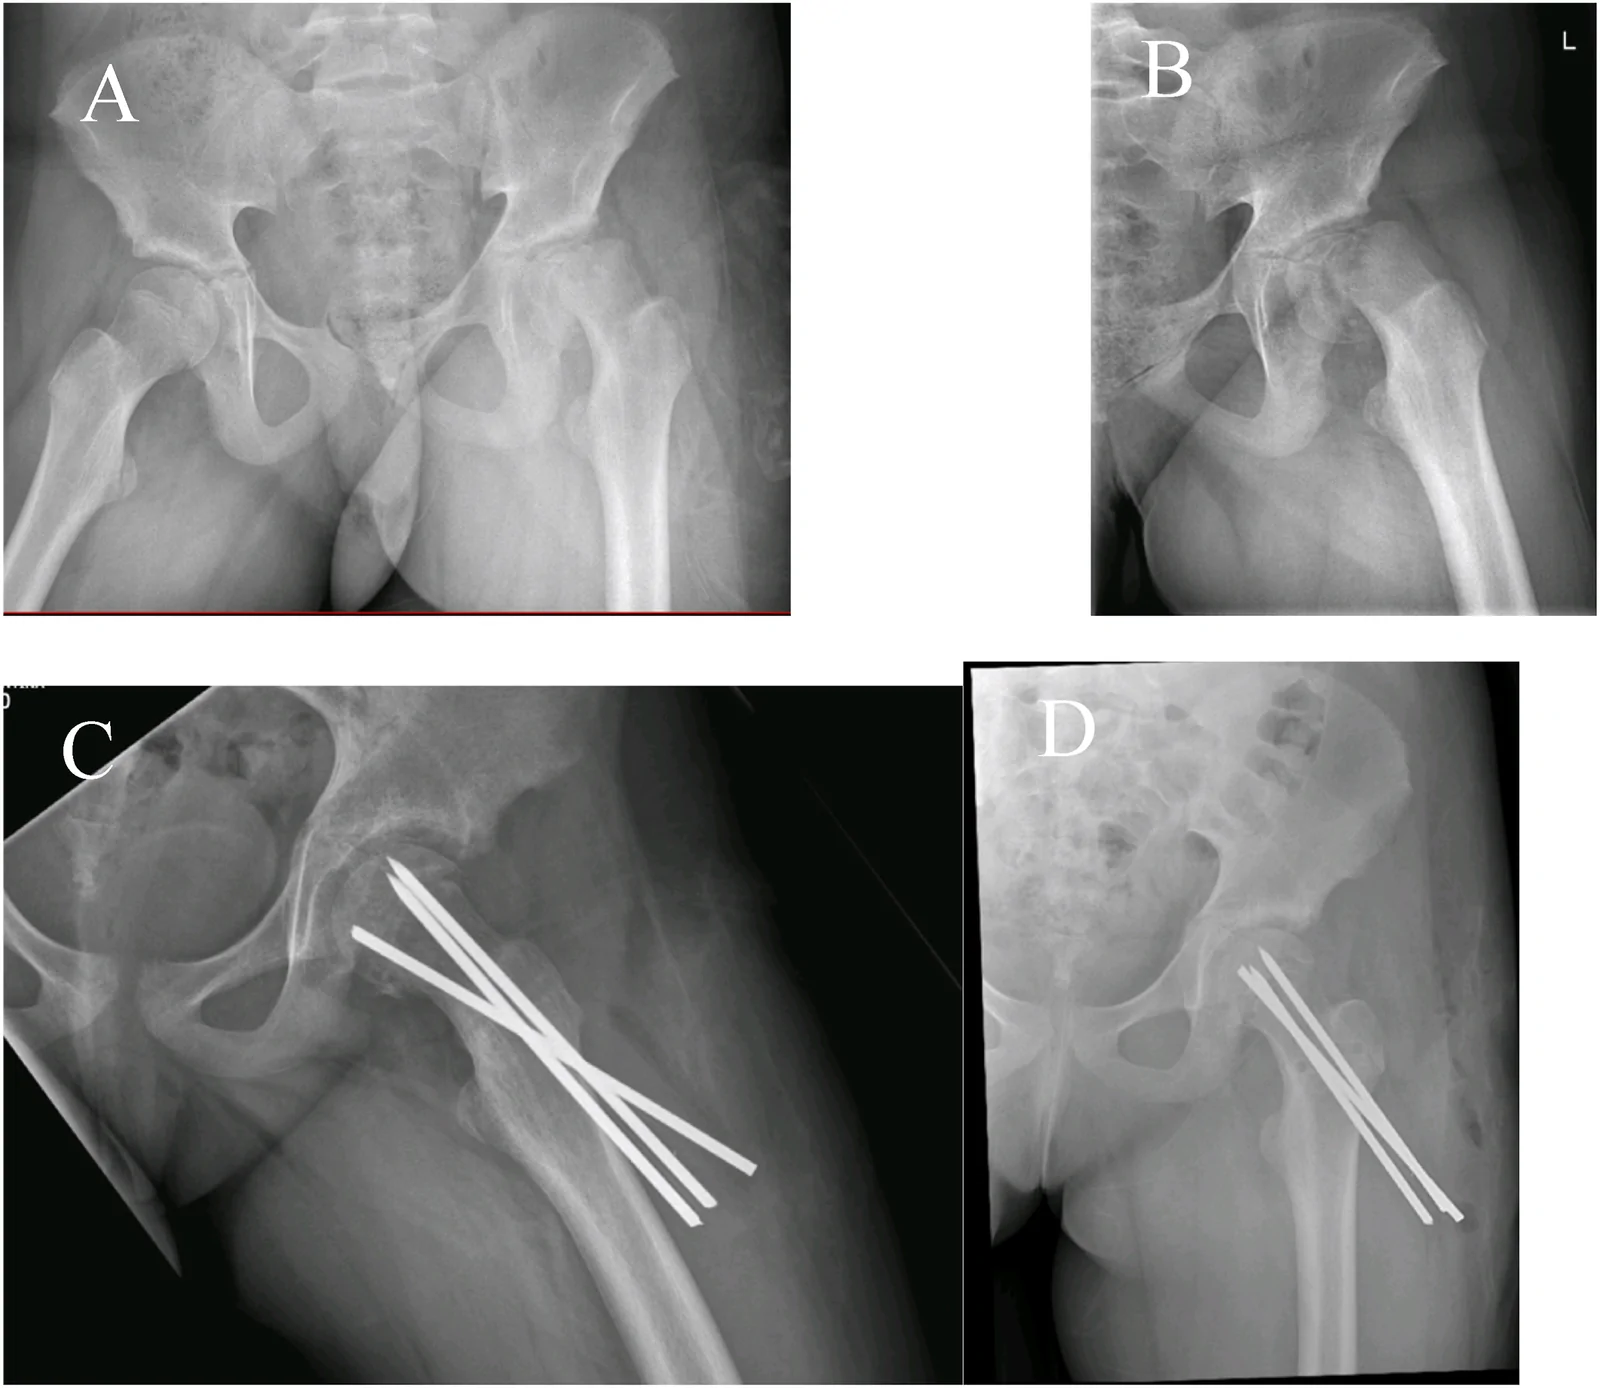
Radiographic images from (A and B) a severe SCFE of the left hip, treated by open realignment through DAA (C and D). Realignment fixation was conducted by three treaded K-wires, inserted from a lateral incision. The K-wires were left prone to ease removal after three months.
